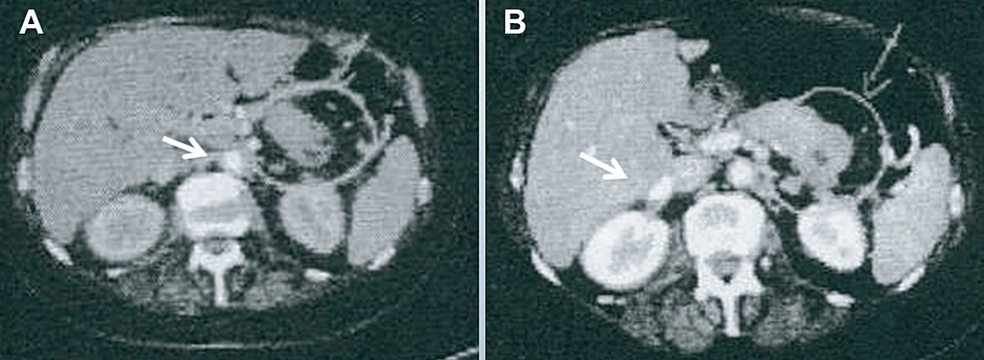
Positron emission tomography (PET)-CT (A) and CT with contrast medium of the abdomen showing enhancement of the corpus and cauda (B) of the pancreas, suggestive of pancreatitis.
